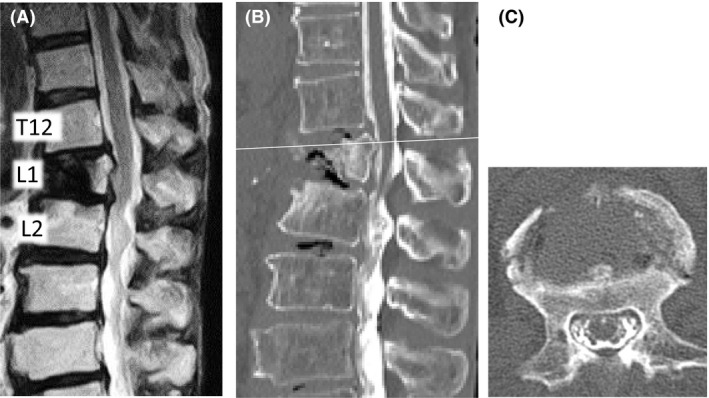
Magnetic resonance imaging and computed tomography after myelography. (A) T2‐weighted magnetic resonance imaging (MRI) of a sagittal section. (B) Computed tomography (CT) of a sagittal section after myelography in the supine position revealed that the termination of the spinal cord was at the upper level of the L2 vertebra. (C) This is shown at the white line on CT of an axial section. (B, C) The spinal canal with space around the cord behind the L1 vertebra.
